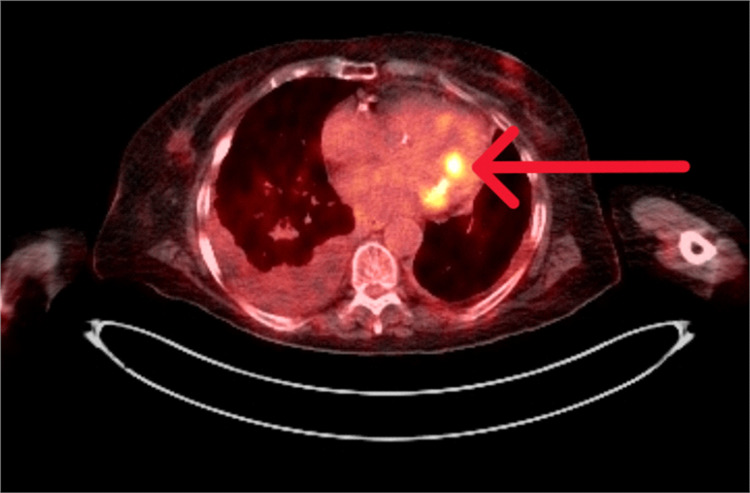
Cross-sectional image of 18F-FDG PET/CT showing increased FDG uptake of mitral valve 18F-FDG, fluorine-18-fluorodeoxyglucose; FDG, fluorodeoxyglucose
